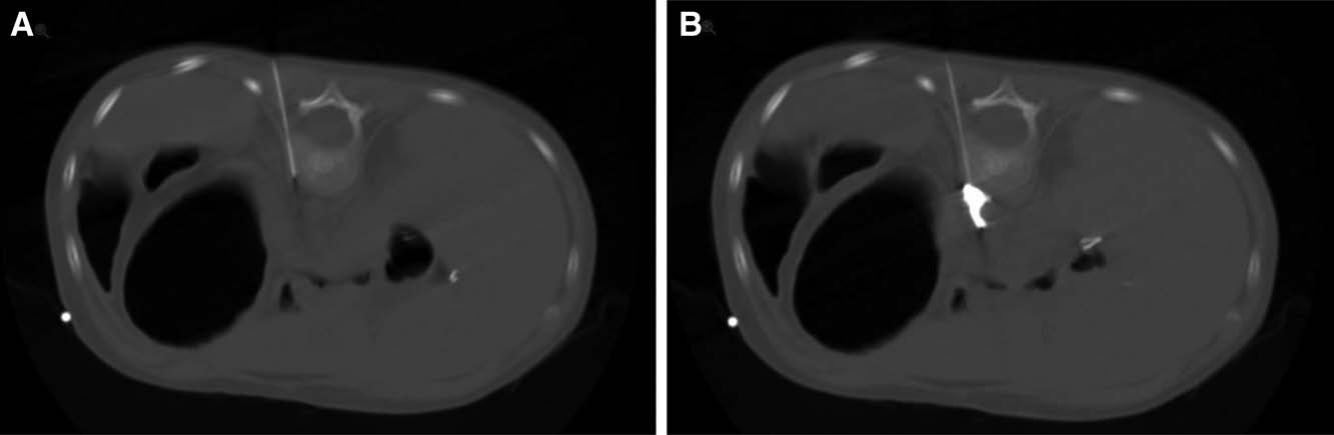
CT-guided posterior lumbar approach to the celiac plexus. A) Direction of approach to the anterior margin of L1 body. B) Contrast dispersion to confirm correct placement of 22G needle in retroperitoneum.
